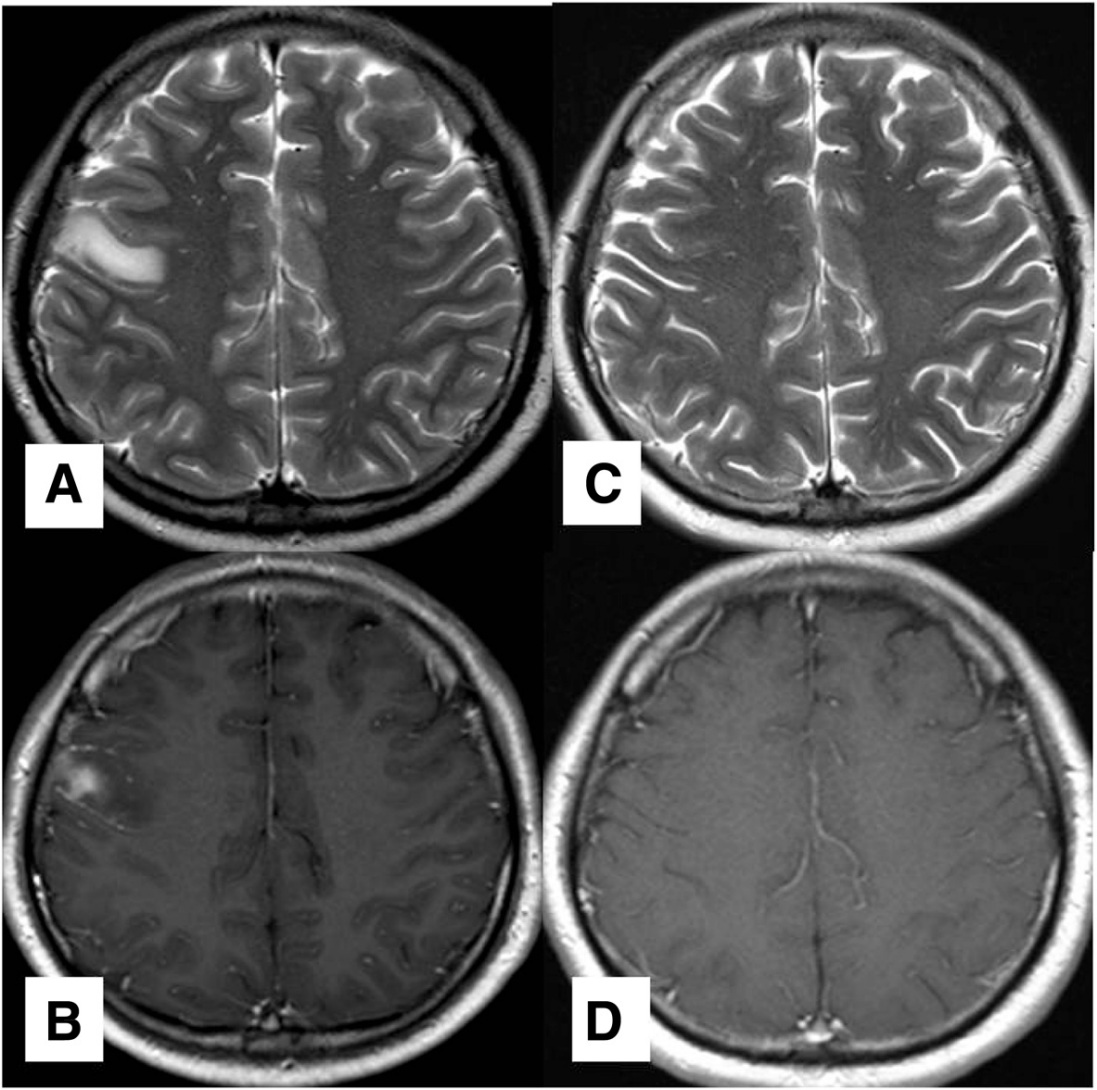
Magnetic resonance imaging of the brain. a-b T2W and T1W with Gadolinium contrast images on September 17, 2013. MRI scan showed hypersignal intensity and gyral contrast enhancement at the right frontal lobe lesion. c-d On February 17, 2014, after steroid initiation, MRI scan showed complete resolution of the right frontal lobe lesion
